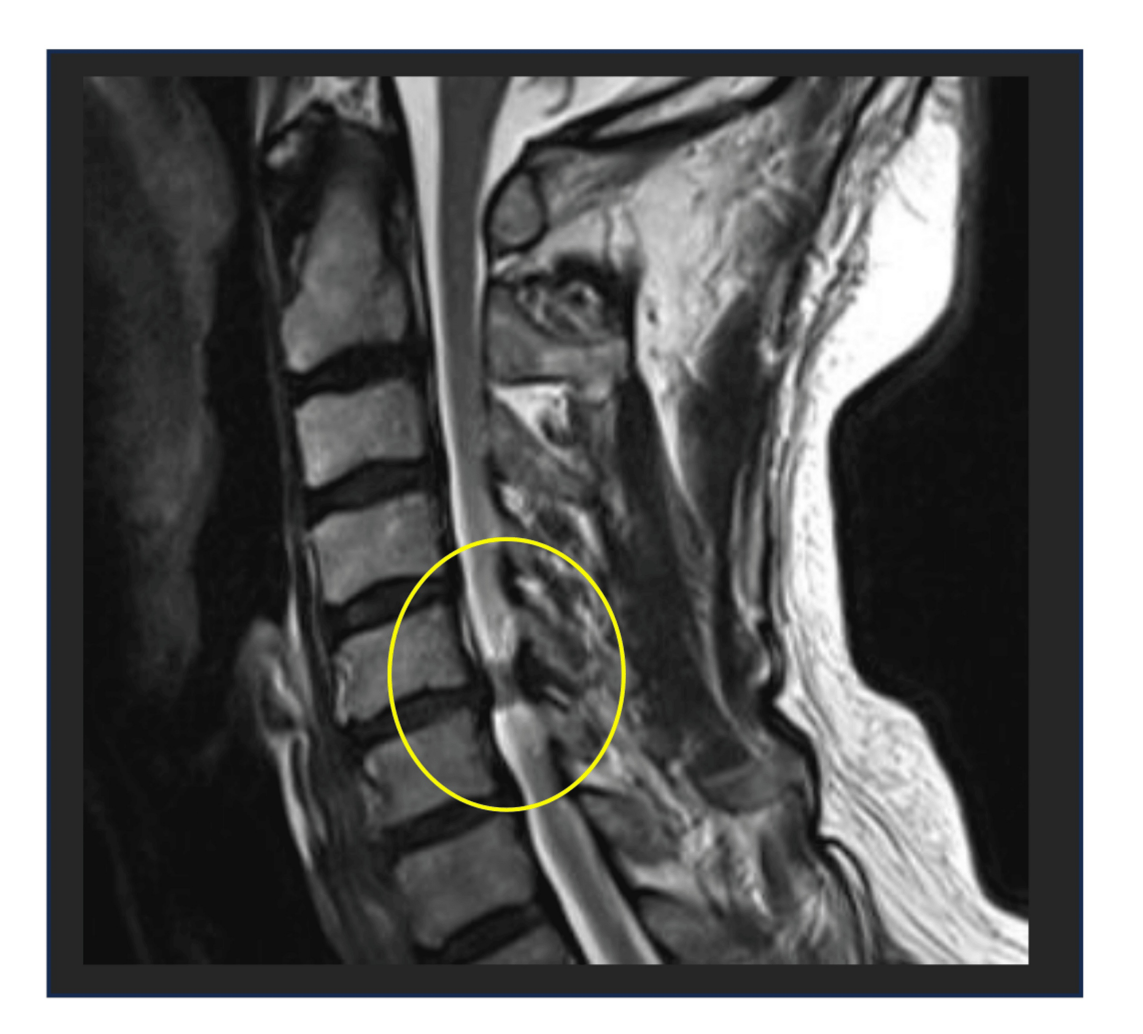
Preoperative T2-weighted MRI of the cervical spine Sagittal T2-weighted MRI showing spinal cord compression at C5/6. MRI, magnetic resonance imaging.
